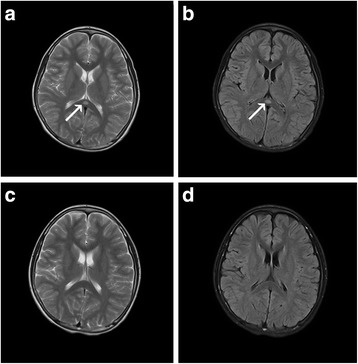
MRI findings of case 2. T2-weighted image (a) and FLAIR image (b) on the day of admission showed a focal high-signal lesion in the splenium of the corpus callosum (*arrow*). On the eighth day after admission, the splenial lesion had completely disappeared on T2-weighted image (c) and FLAIR image (d)
